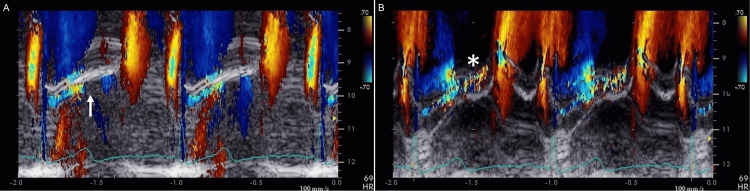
Echocardiography before and after leg elevation Color-Doppler M-mode images obtained in the left lateral decubitus position show that the mosaic signal of mitral regurgitation ends in the mid-systole before an elevation of the legs (A, arrow). After the leg lift, the mosaic signal extends to the end of the systole or continues until the start of the left ventricular inflow (B, asterisk).
